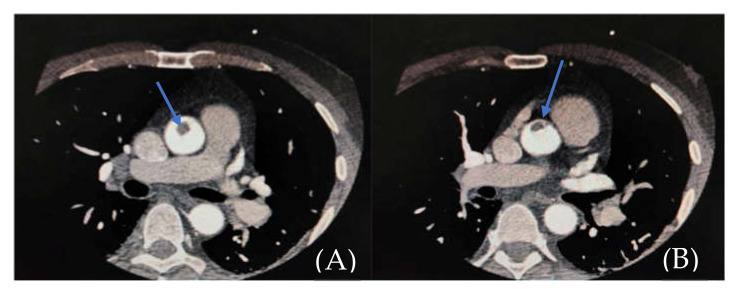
(A,B). CTA showing endoluminal aortic thrombus (filling defect in the ascending aorta—blue arrow).
